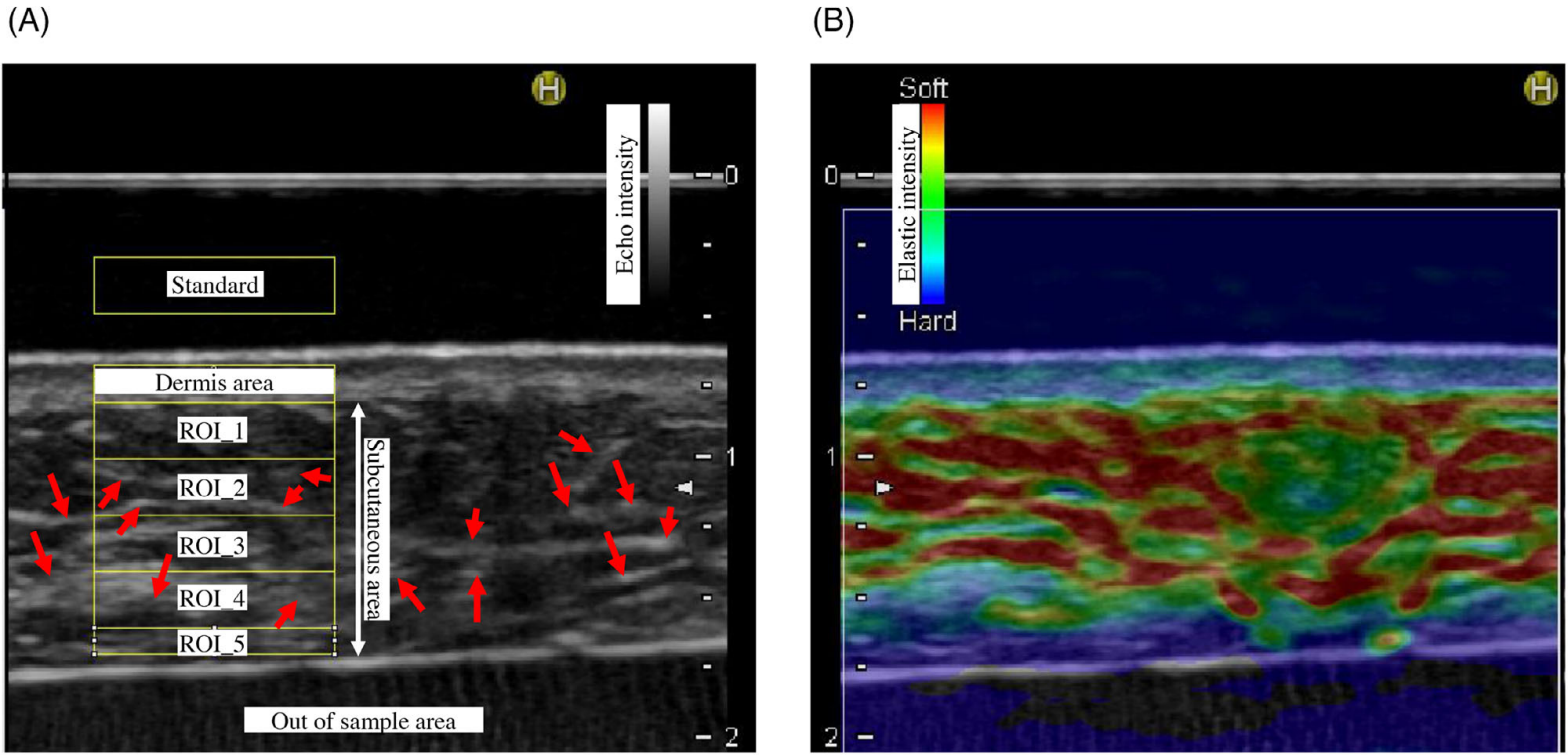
Typical examples of ultrasound and ultrasound elastography images. (A) Setting the analysis area performed on an ultrasound image. A region of interest (ROI) with a thickness of 0.23 mm and a constant width was set to be tangential to the border region between the dermis and subcutaneous fat layer (ROI_1). The ROI was set to the lower edge of the tissue using the same method. The red arrows indicated fiber structures across the subcutaneous tissue. (B) Ultrasound elastography image of the same area as in (A).
